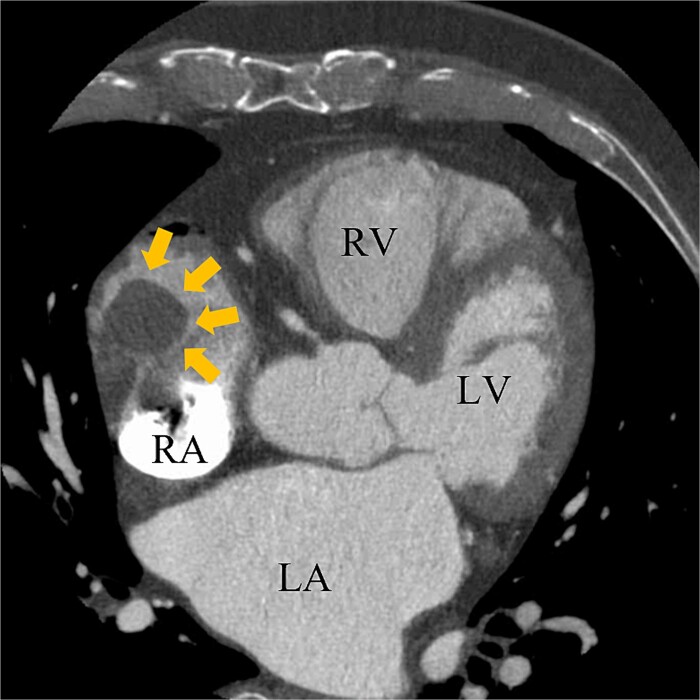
Preoperative enhanced computed tomography imaging showing a 25-mm intra-cardiac mass in the RA (arrows). RV, right ventricle; LA, left atrium; LV, left ventricle.
